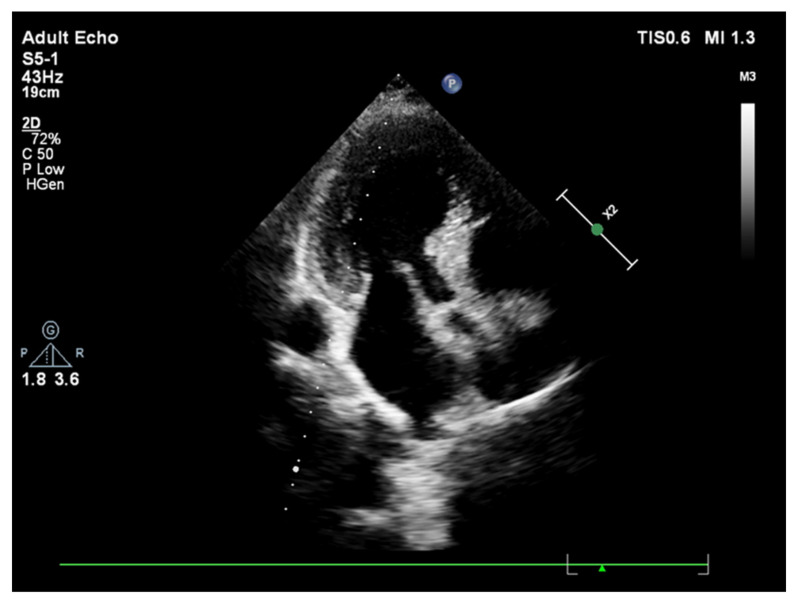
A sharp curvature of more remarkable basal septal hypertrophy from apical 4 chamber view during end-diastole in another hypertensive patient.
